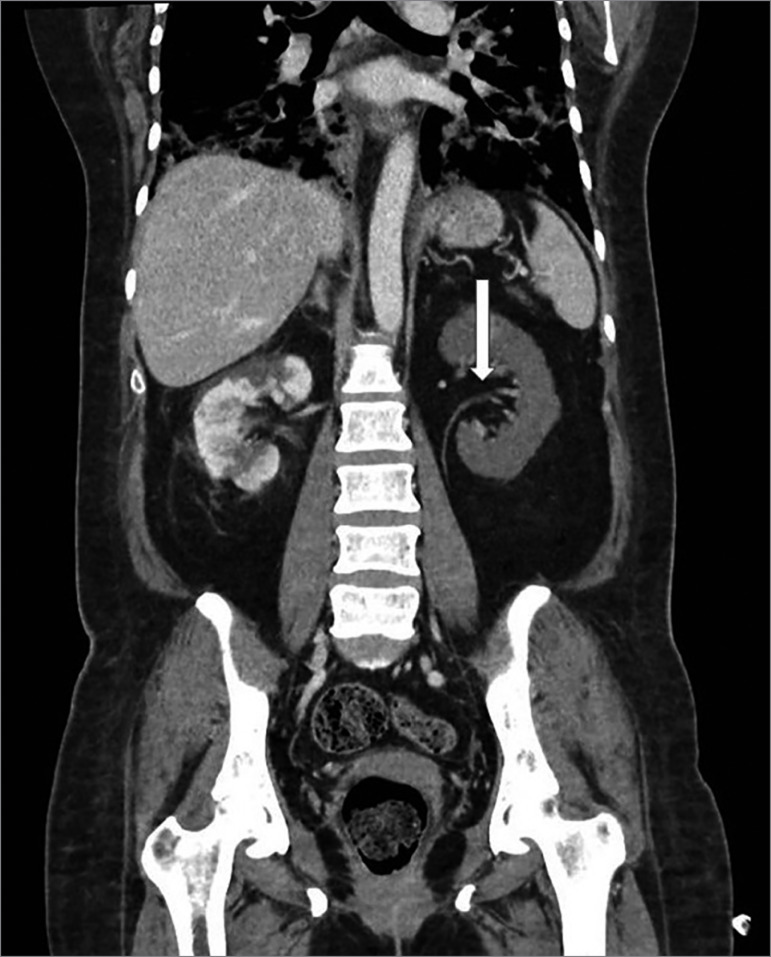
Abdominal computed tomography showing thrombus in left kidney artery immediately before its bifurcation. The thrombus totally obstructs its previous division and partially obstructs the posterior division.
